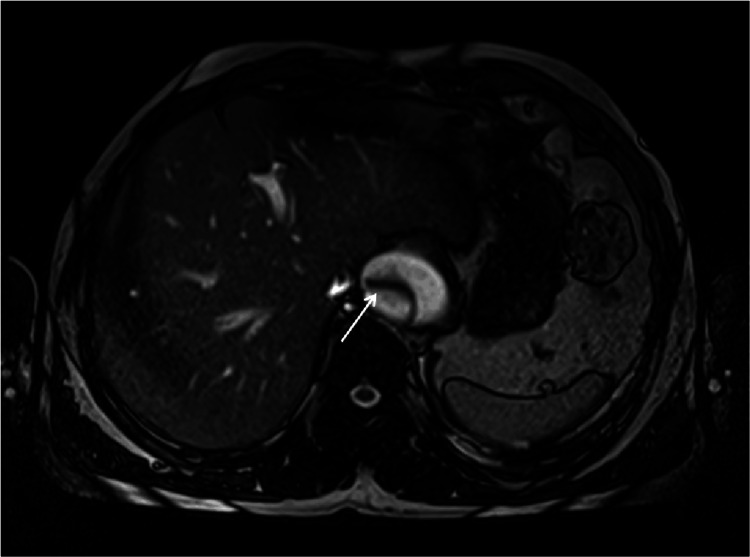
T2-weighted MRI - axial section at the level of liver highlighting an aortic dissection
